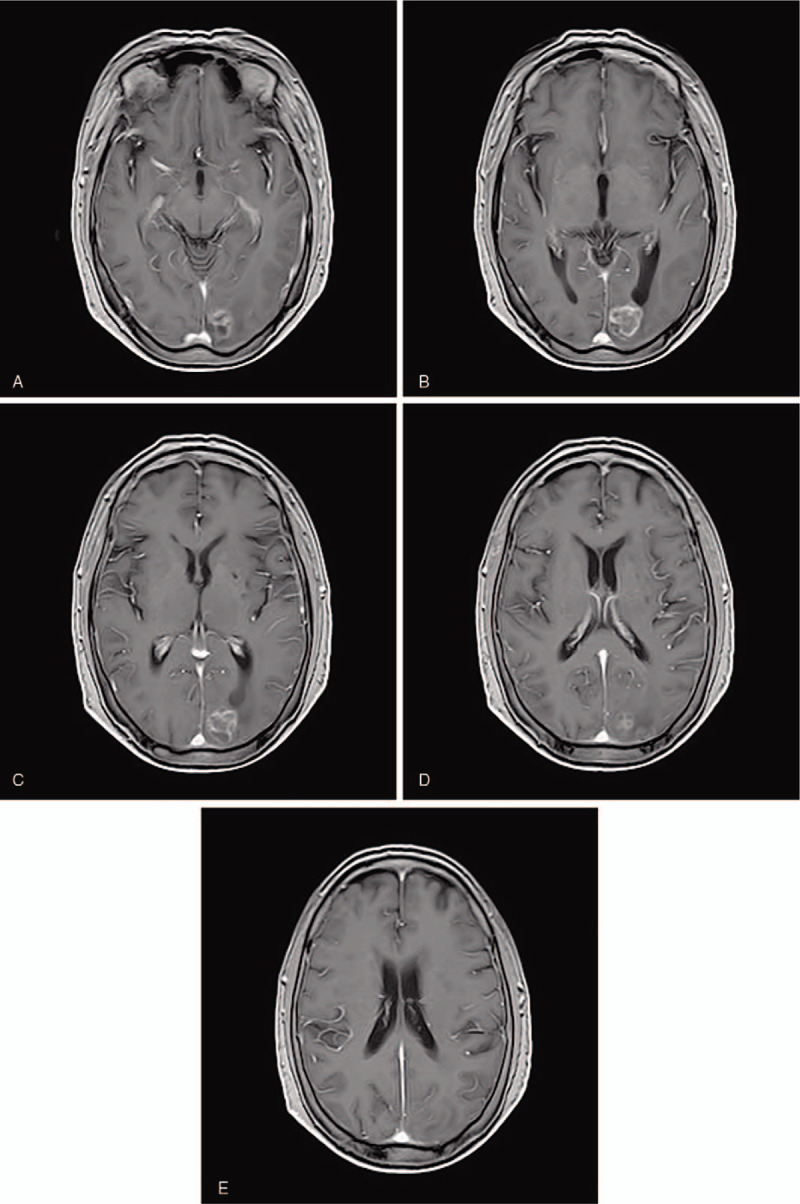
(A–E) Magnetic resonance imaging of left occipital metastasis 1 mo after radiotherapy.
